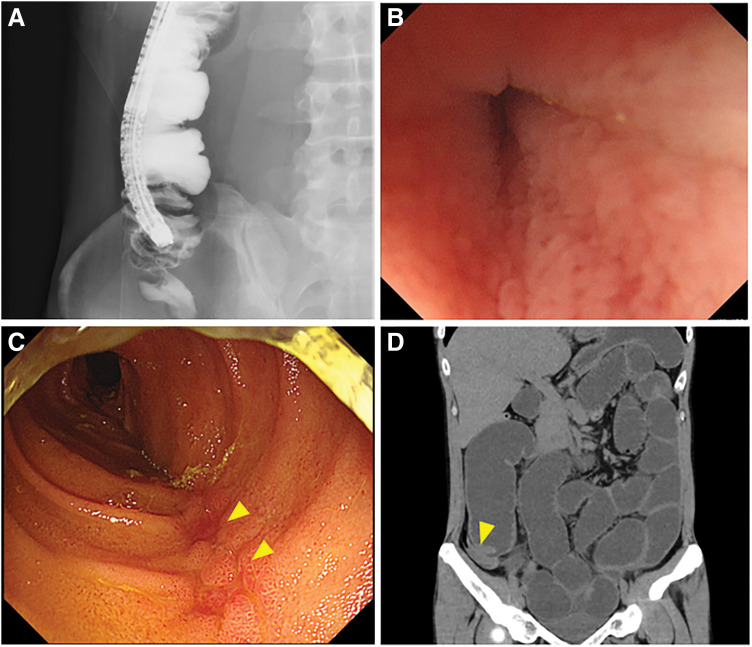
Preoperative findings. (A) Site of stenosis identified by gastrointestinal contrast imaging. (B) Severe stenosis at the ileocecal region. (C) Longitudinal ulcer in the terminal ill proximal to the stenotic segment (arrowheads). (D) Preoperative CT scan of severe stenosis (arrowhead).
